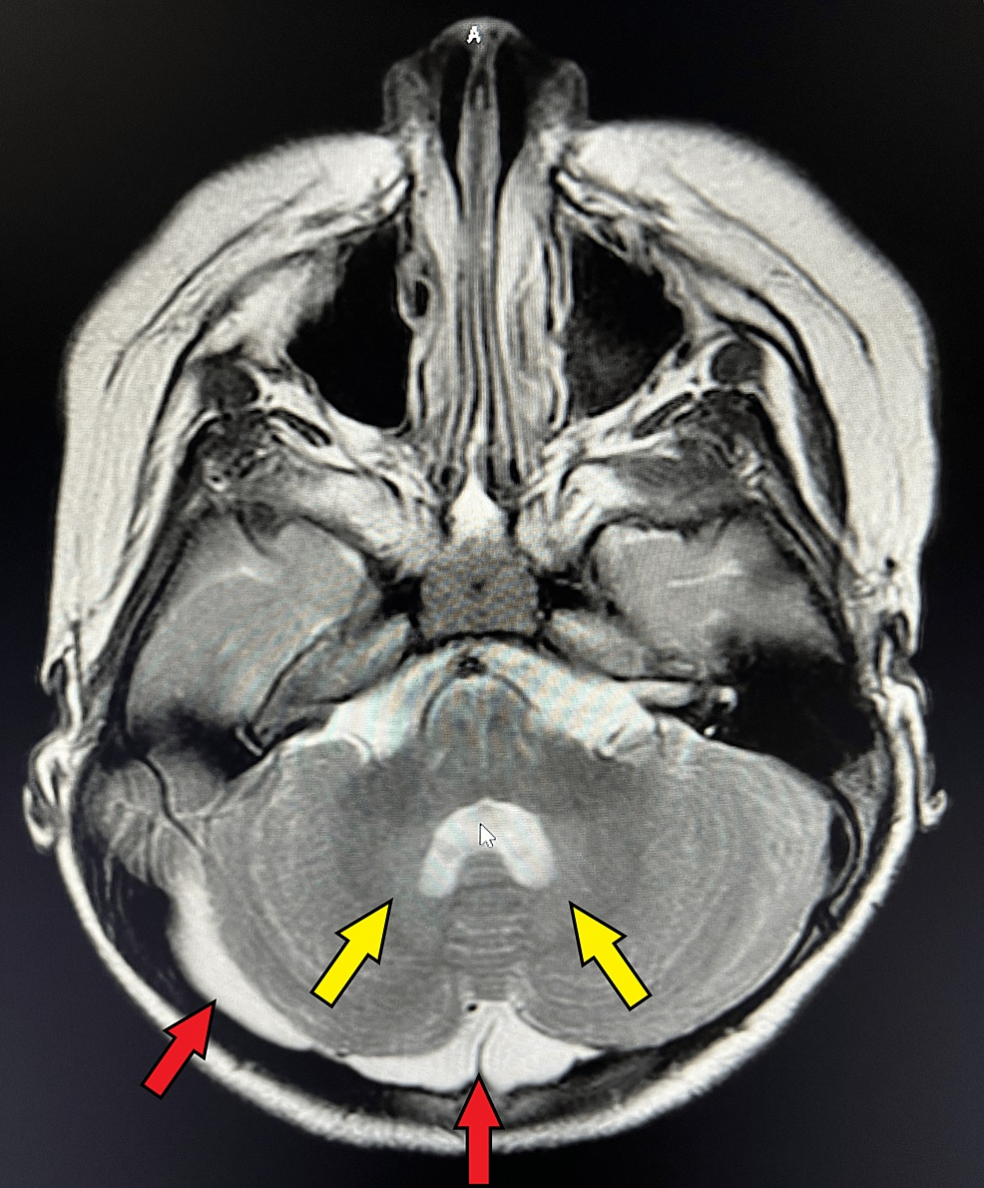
T2-weighted axial MRI A T2-weighted image displays mild cerebellar dysplasia along with a notable signal within the dentate nuclei (yellow arrows). Additionally, collections of cerebral spinal fluid are visible peripheral to the right cerebellar hemisphere and posterior to the cerebellar vermis (red arrows). These may be arachnoid cysts.
